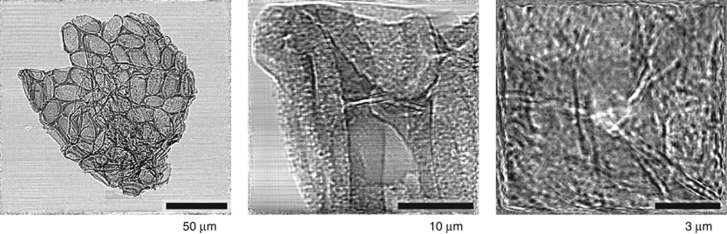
In-line projection holograms of an Acantharian cyst obtained at the PETRA III P11 beamline using 16.3 keV X-rays. The magnification is increased as the sample is moved towards the X-ray focus. The exposure time of the first image was 2 s, over which time 1.7 × 10^9^ photons were recorded. The exposure time of the other images was 5 s, with 3.7 × 10^9^ detected photons.
